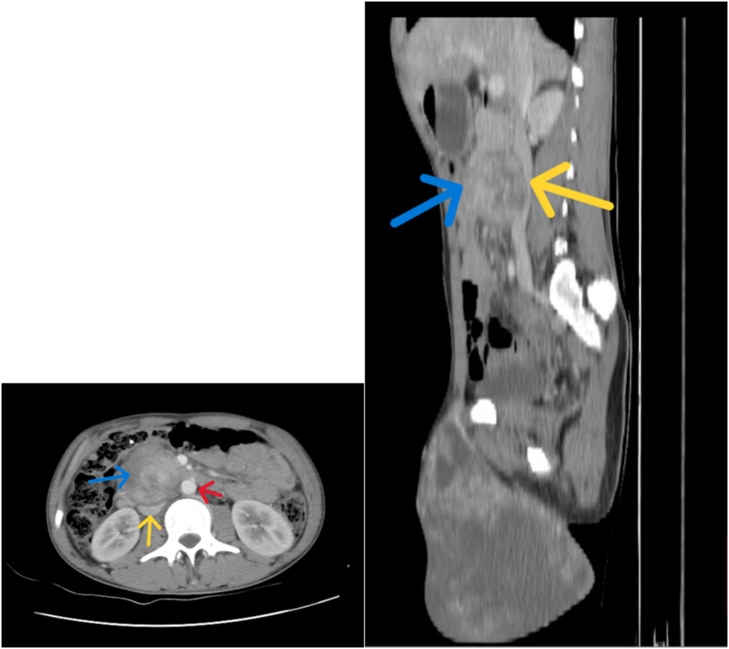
Contrast enhanced CT scan images of the abdomen depicting a heterogeneously enhancing retroperitoneal lymph node (blue arrows) pushing between the abdominal aorta (red arrow) and inferior vena cava (yellow arrows).
